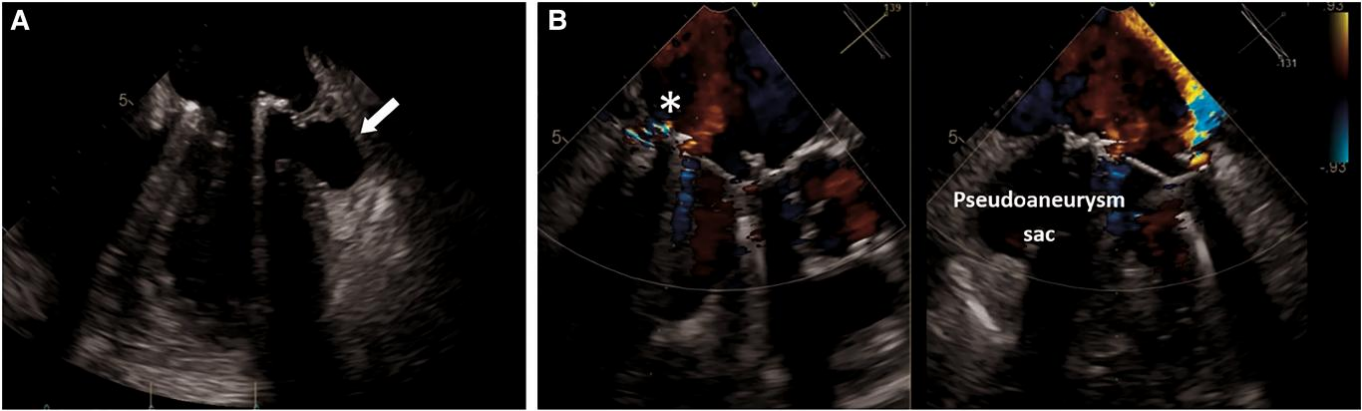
(*A*) Mid-oesophageal four-chamber view showing pseudoaneurysm arising from the basal postero-lateral wall of the left ventricle. (*B*) X-plane mid-oesophageal long-axis view: colour Doppler shows the flow through the cavity between the left atrium and left ventricle (arrows).
